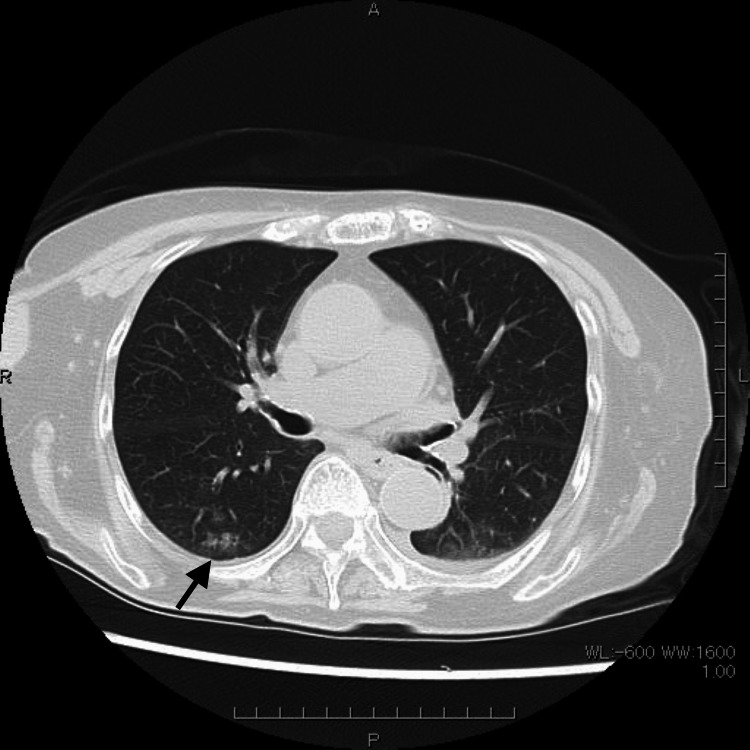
Plain chest CT Plain chest CT showing a faint area of ground-glass opacity (arrow) in the upper segment of the right lower lobe, consistent with early COVID-19 pneumonia
